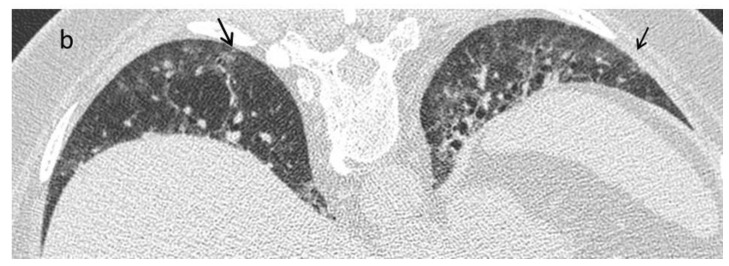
Early stage. A 42-year-old female with a diagnosis of SSc. (a) Axial High Resolution CT image shows subtle ground glass opacities (black arrows) in the peripheral areas of lung bases. An additional scan with patient in prone position can be helpful to differentiate gravity-related interstitial alterations from disease-related alterations. (b) The persistence of ground-glass opacities in the prone position is related to a nonspecific interstitial pneumonia (NSIP) pattern.
